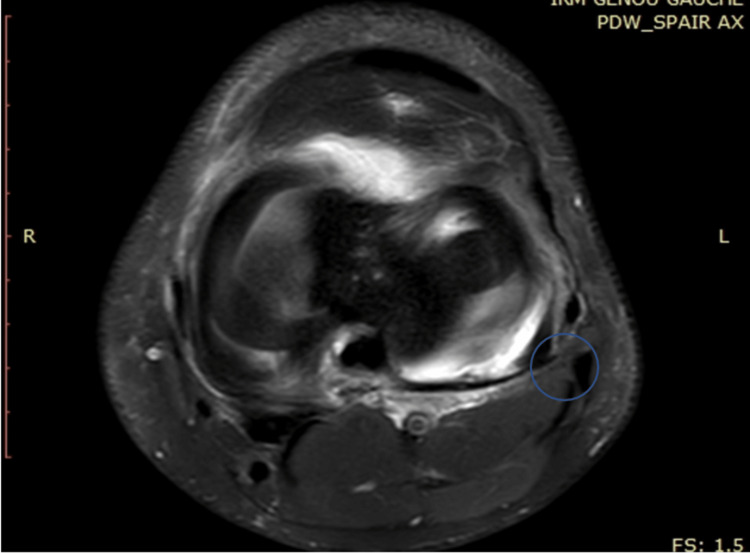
MRI showing the proximity of the semimembranosus tendon to the medial meniscus
